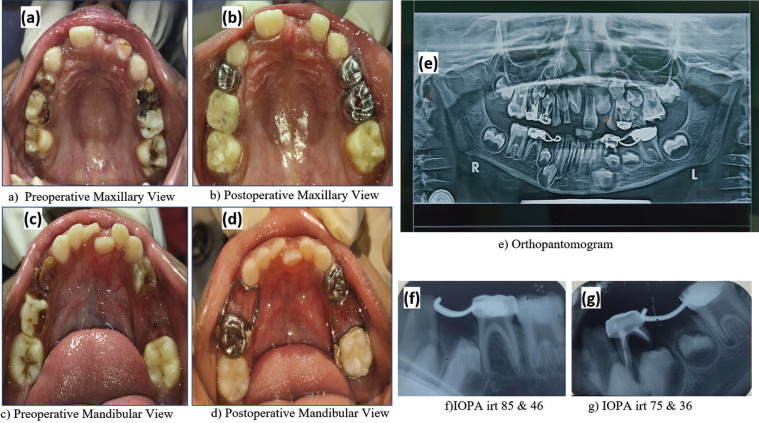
Intraoral pictures along with radiographs (a) Preoperative Maxillary View, (b) Postoperative Maxillary View, (c) Preoperative Mandibular View, (d) Postoperative Mandibular View, (e) Orthopantomogram, f) IOPA irt 85 & 46, g) IOPA irt 75 & 36.
